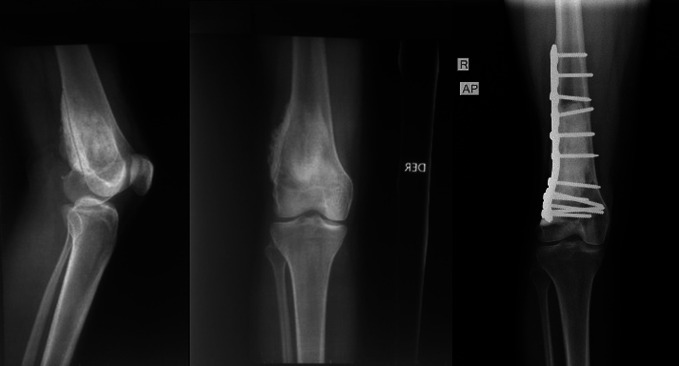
AP and lateral radiographs of the right knee showing a distal femur parosteal osteosarcoma. AP radiograph of the right knee after resection and reconstruction.
